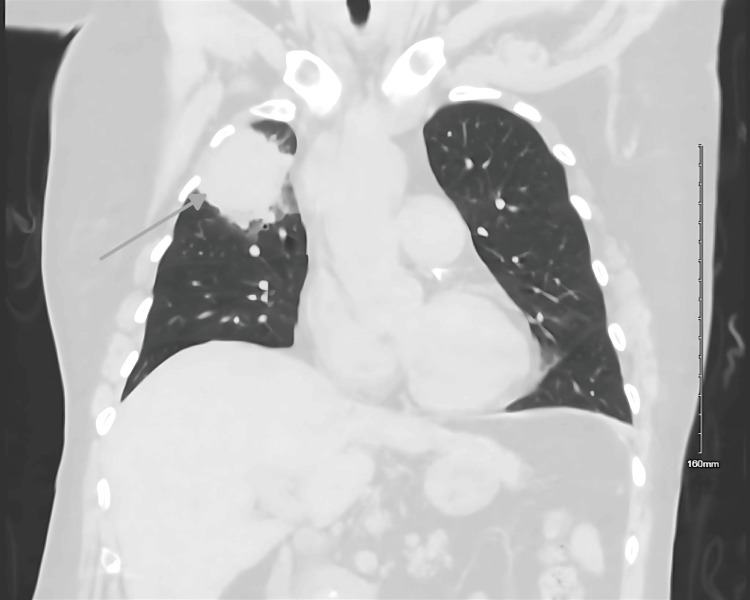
Computed tomography scan of the chest showing a large anterior right upper lobe masslike consolidation (gray arrow)
